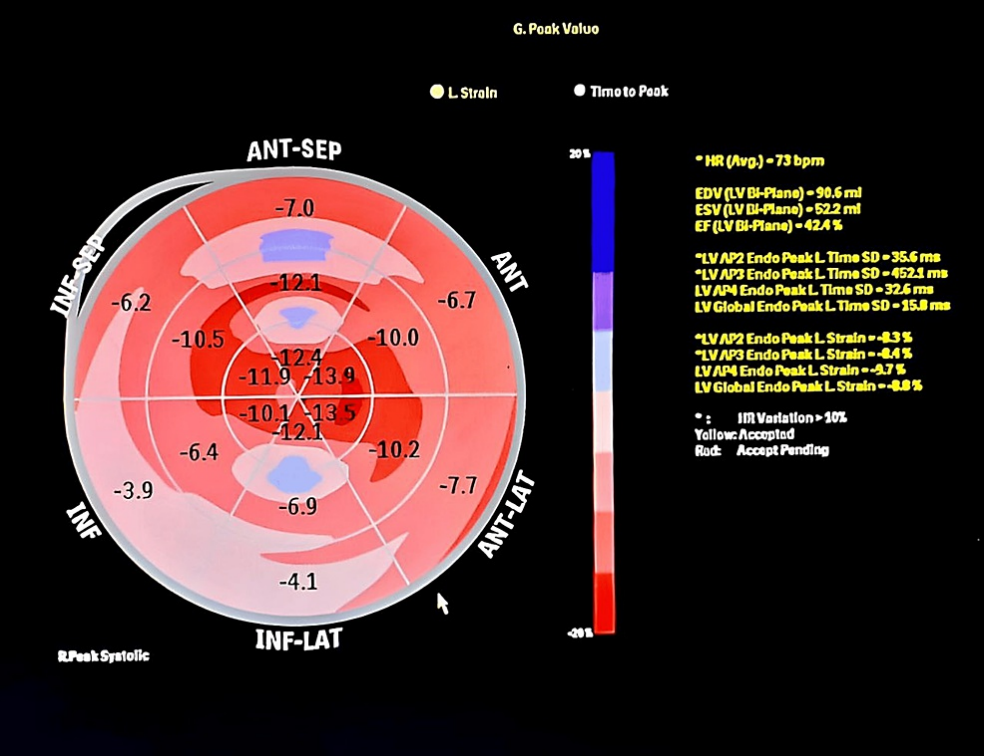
Two-dimensional speckle tracking echocardiography bull’s eye plot showing left ventricular strain and apical sparing.
